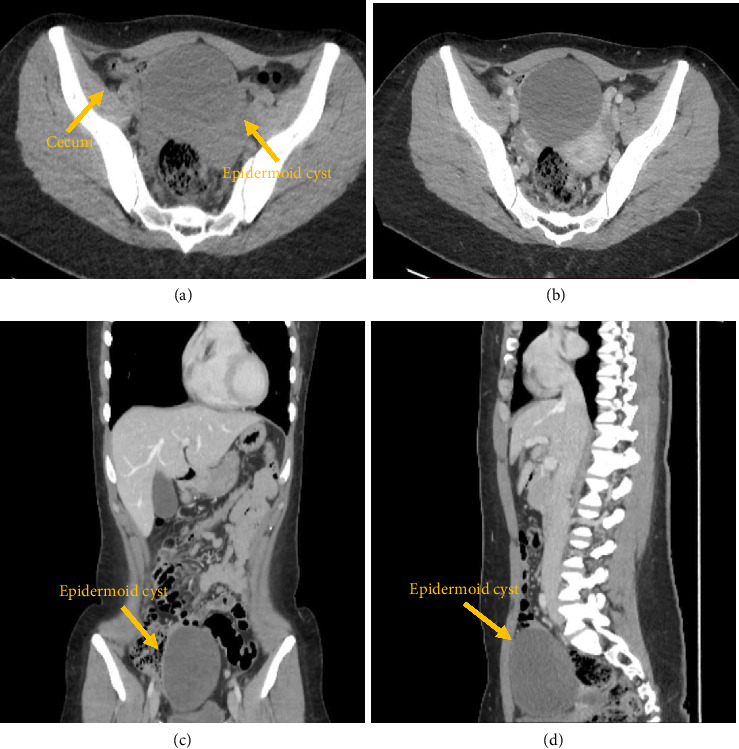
Contrast-enhanced CT. Computed tomography scan image showing a well circumscribed cystic mass adherent to the cecum and containing heterogeneous content of increased attenuation (a–d). (a) Axial CT image before contrast. (b) Axial CT contrast-enhanced image. The wall shows enhancement. (c) Coronal CT contrast-enhanced image shows the craniocaudal extension of the mass. (d) Sagittal CT contrast-enhanced image shows the anteroposterior extension of the mass.
